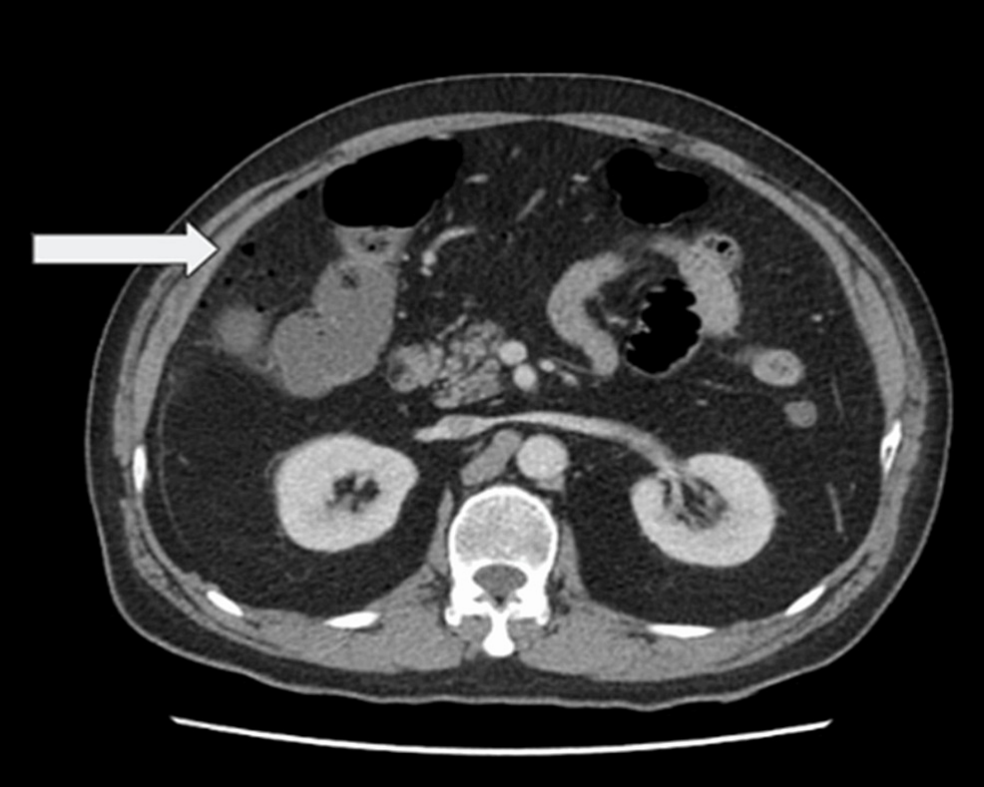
Abdominal CT scan showing free air specks in the abdominal cavity CT: Computerized tomography
